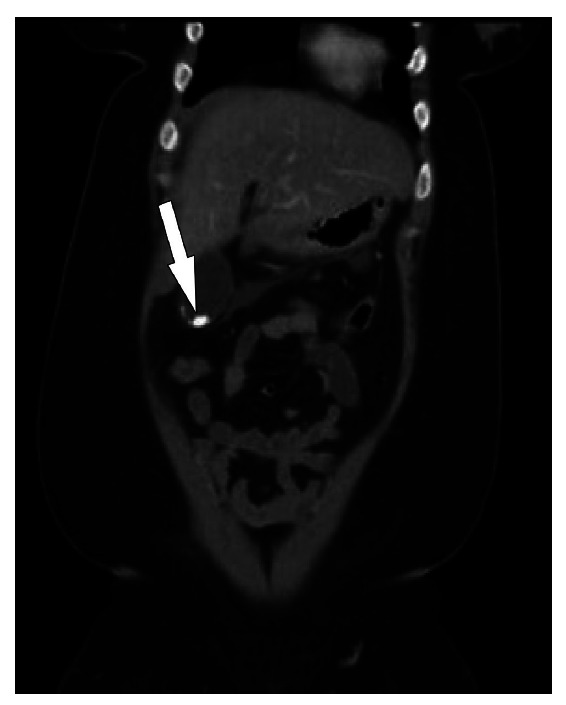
Computed tomography scan of a patient with gallstones (arrow).
